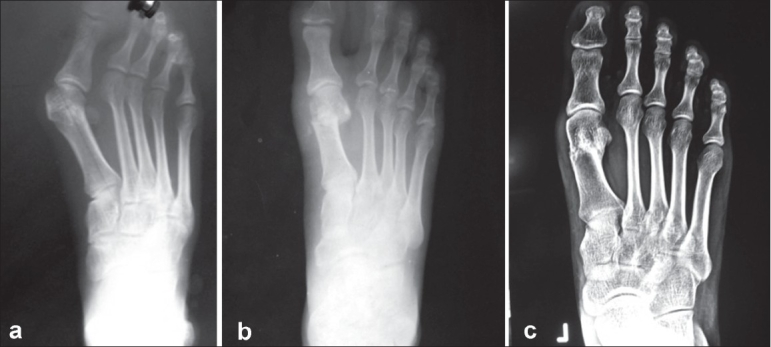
(a) Anteroposterior radiograph of left foot shows hallux valgus angle of 40°, (b) Radiograph at 4 months follow-up showing correction achieved, (c) Radiograph at 6 months showing the correction achieved and union at the osteotomy site.
